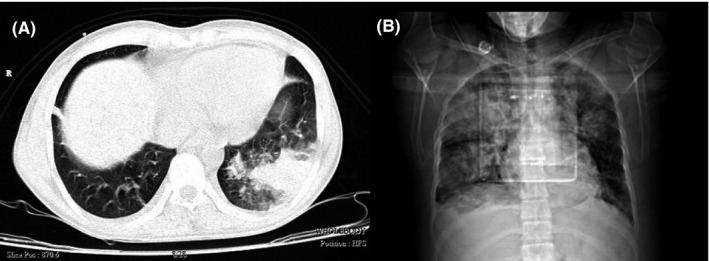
Computed tomography (CT) scans of the chest suggestive of COVID‐19. Ground‐glass opacities patch and consolidation patch in the peripheral areas of the lobes of the lungs (A), multiple consolidation and confluent lesions and organic pneumonia (B)
